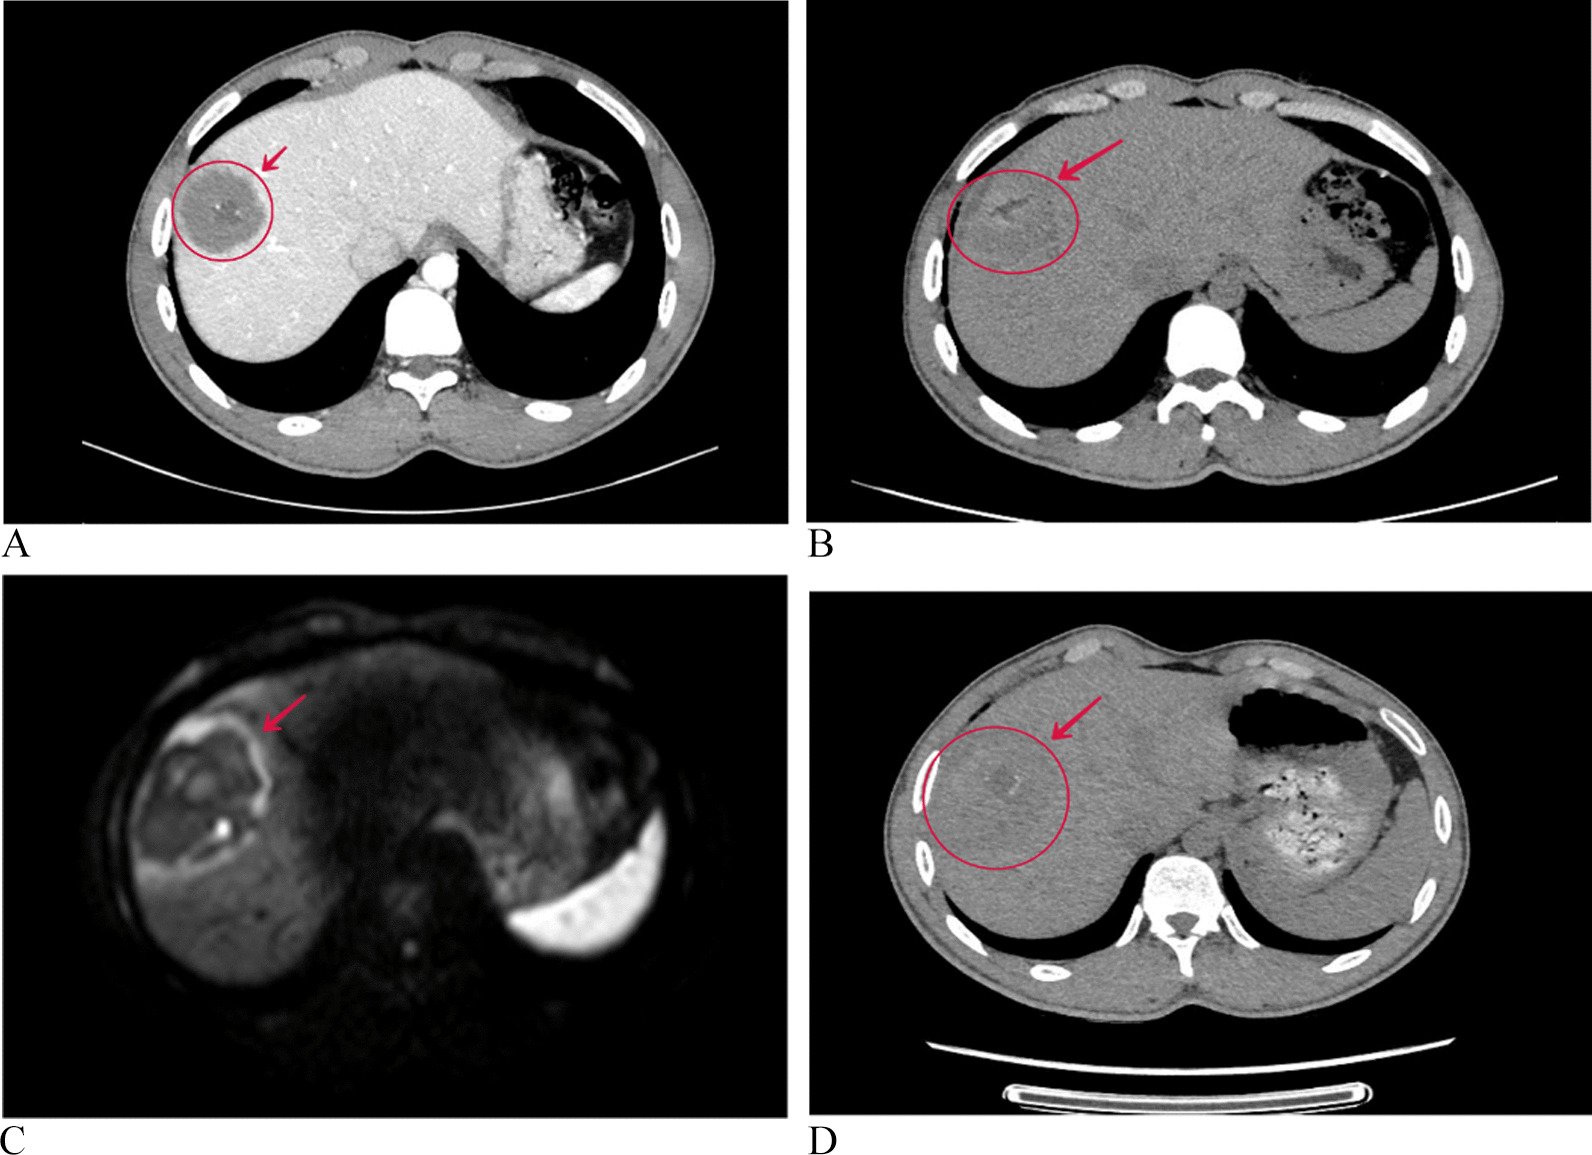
Images showing a lesion in an 18-year-old male patient with HAE recurrence before and after MWA treatment. A Enhanced CT was performed before the first ablation, and the lesion was marked by the red arrow. B CT scan on the first day after the first ablation, suggesting complete ablation. C At 17 months after MWA treatment, MRI-DWI suggested that the diffusion was limited around the lesions, suspected to be HAE recurrences. D CT scan on the first day after the second ablation suggesting complete ablation. HAE, hepatic alveolar echinococcosis; MWA, microwave ablation
